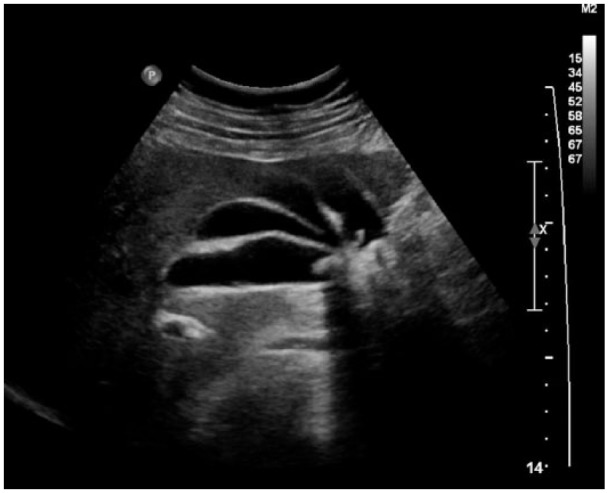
Ultrasound: multiple junctional folds, cholelithiasis. Gallbladder is borderline enlarged.
